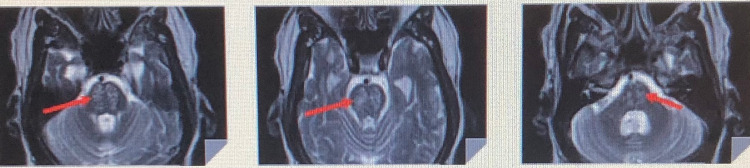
Patient magnetic resonance imaging showing findings leading up to diagnosis Red arrows showing the patchy diffusion restriction with associated T2/fluid-attenuated inversion recovery.
